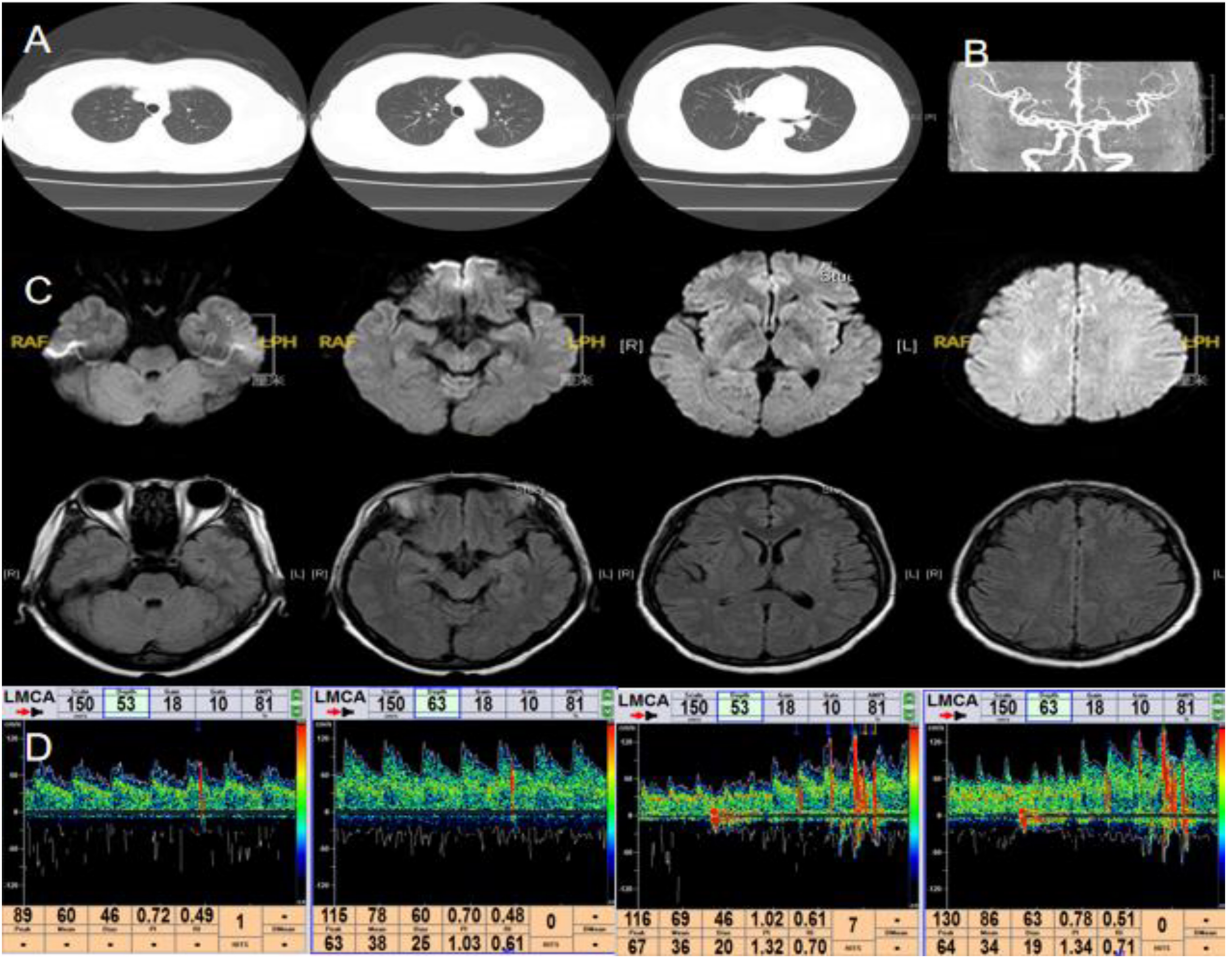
Main examination findings for case 2: (A) chest CT; (B) cranial MRA; (C) cranial MRI; (D) transcranial Doppler ultrasound bubble test.
